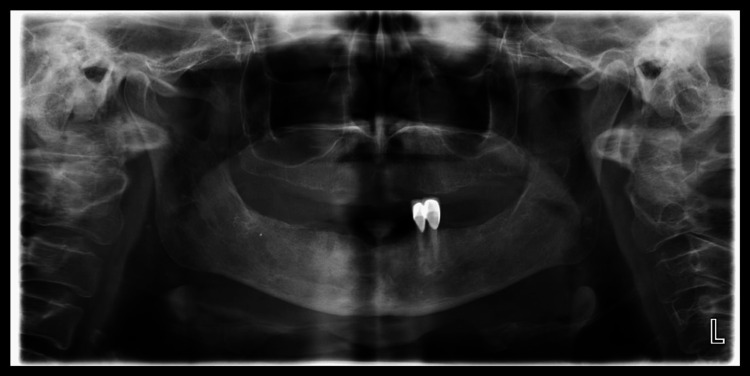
OPG examination of the patient. OPG: Orthopantomography
